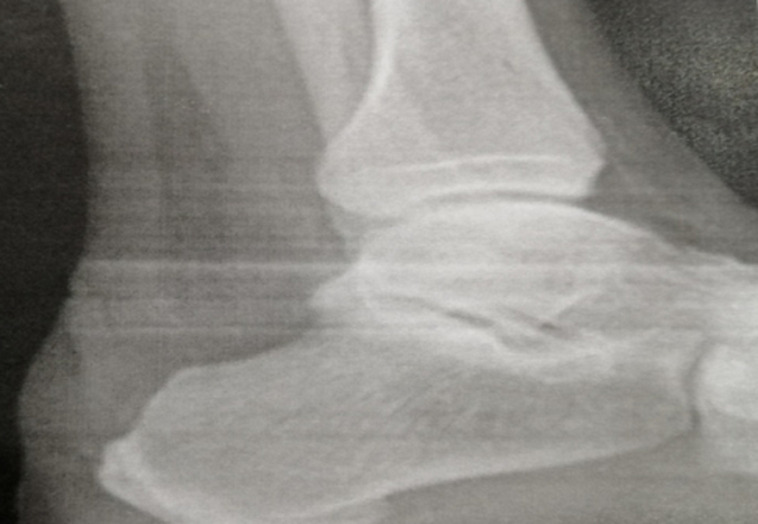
image radiographique par amplificateur de brillance après résection de l´exostose osseuse du calcanéum
